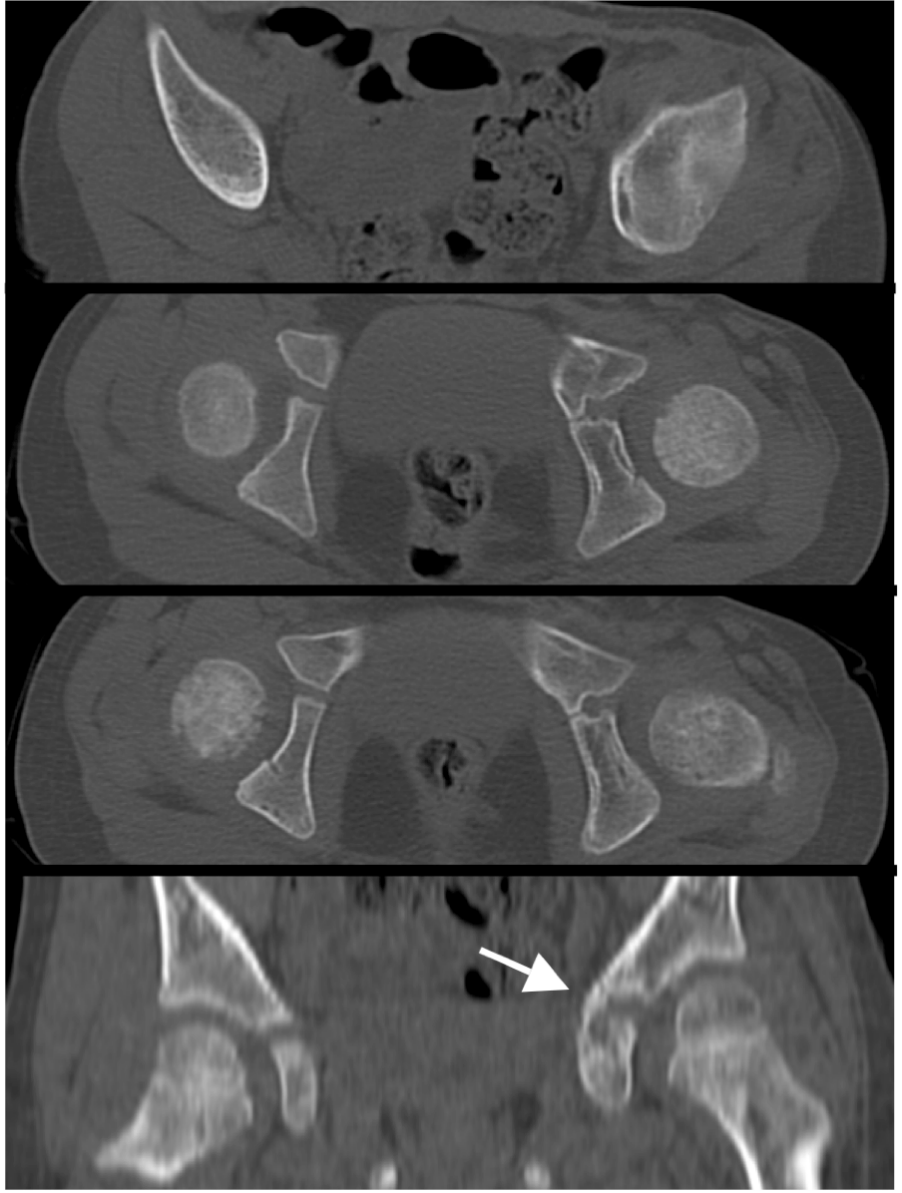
A CT scan obtained after hardware removal at 10 months demonstrates the healed fracture in anatomic position and confirms the suspected early physeal closure of the left acetabulum (arrow in the coronal view).
